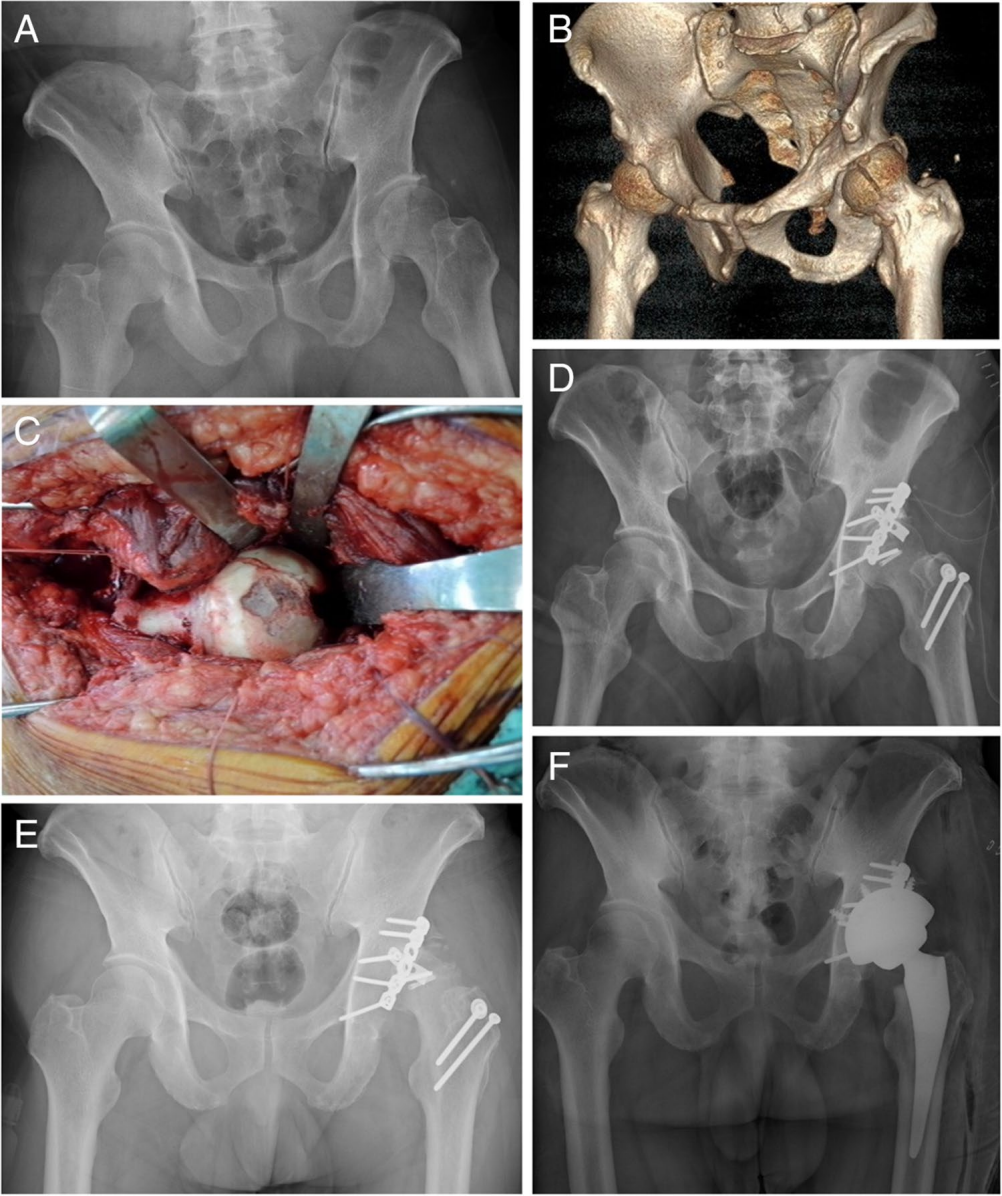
Representative case of a 53-year-old man involved in a motorbike accident. A Left hip Pipkin type IV femoral head fracture of a 53-year-old male after a motorbike accident. B 3D CT images demonstrating displaced femoral head and acetabular posterior wall fracture. C Intra-operative photograph showing femoral head impaction and suprafoveal fracture. D Post-operative radiograph after the patient was operated through trochanteric flip osteotomy. E Seven months after the index surgery, trochanteric osteotomy healed and left femoral head osteonecrosis was noted. F Total hip replacement was performed 11 months after the index surgery. CT, computed tomography, 3D, three dimensional
